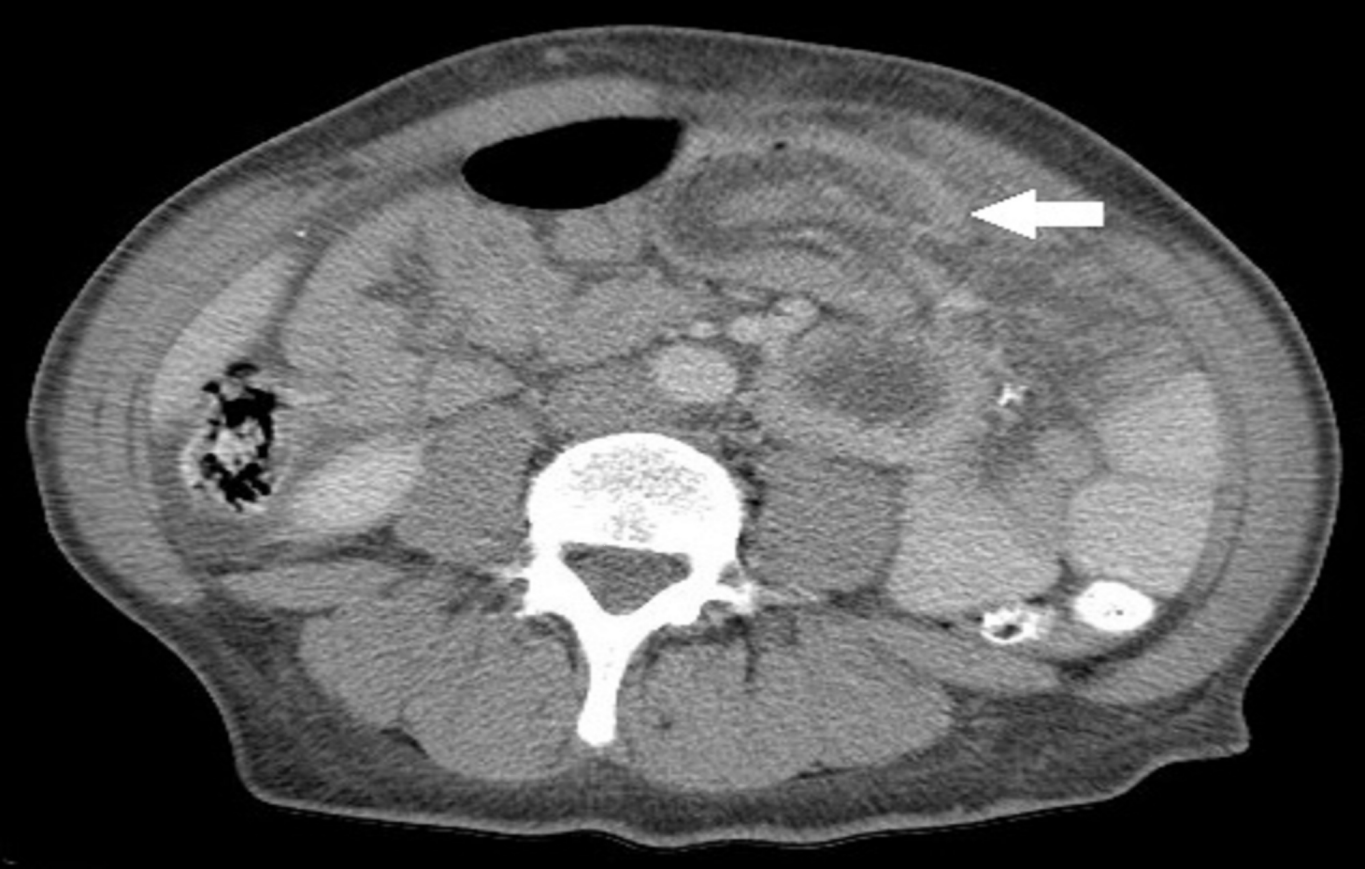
Abdominal computed tomography (CT) scan, axial view, showing dilatation of the biliopancreatic limb of the REYGB with SB intussusception (arrow) through the jejunojejunostomy REYGB: Roux-en-Y gastric bypass surgery; SB: small bowel.
